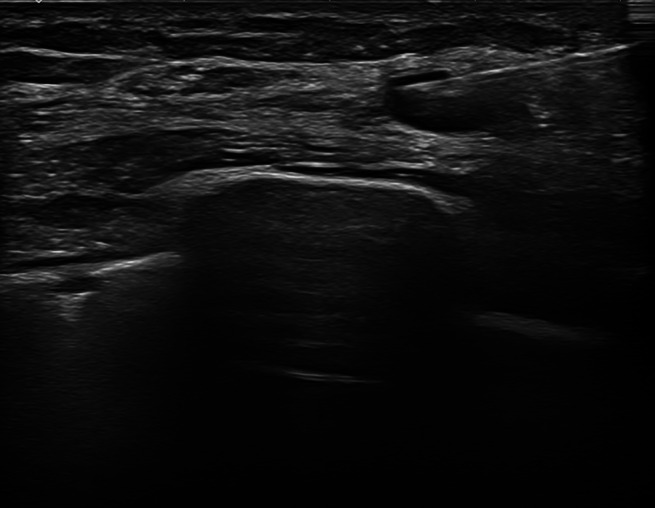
Ultrasound‐guided fine‐needle aspiration cytology of a regional lymph‐node melanoma metastasis to the axilla.
